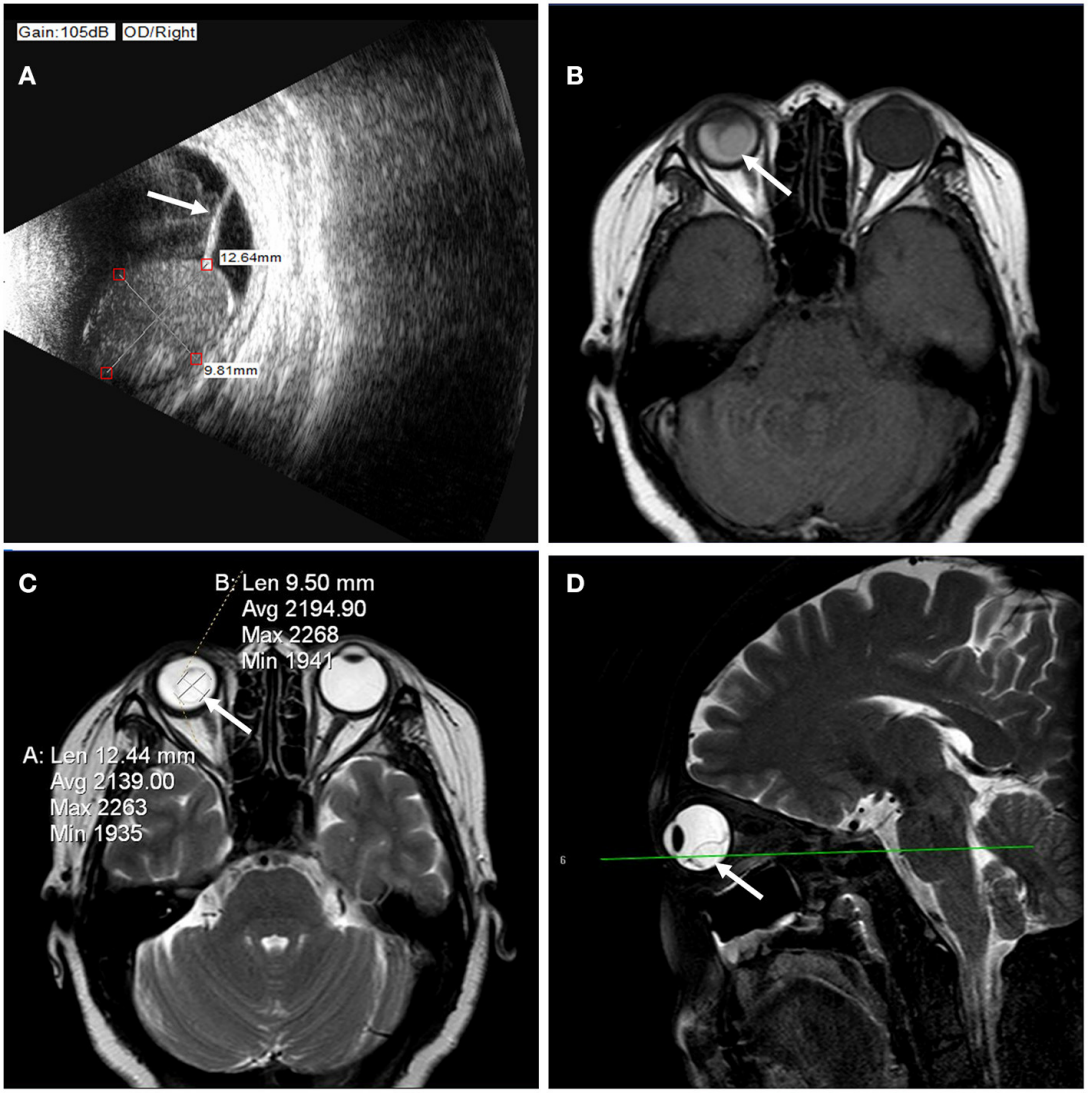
(A) B-ultrasound images showed an intraretinal macrocyst and a detached retina (arrow). The size of the intraretinal macrocyst was approximately 12.64 × 10.42 mm. (B) Orbital MRI revealed a moderate-enhancing mass (arrow) in T1 images in the vitreous cavity of the right eye. (C) Orbital MRI revealed a hyper-enhancing mass (arrow) in T2 images in the vitreous cavity of the right eye. The hyper-enhancing mass was approximately 12.44 × 9.50 mm. (D) The lesion (arrow) was located in the posterior lower part of the vitreous cavity on sagittal scans.
